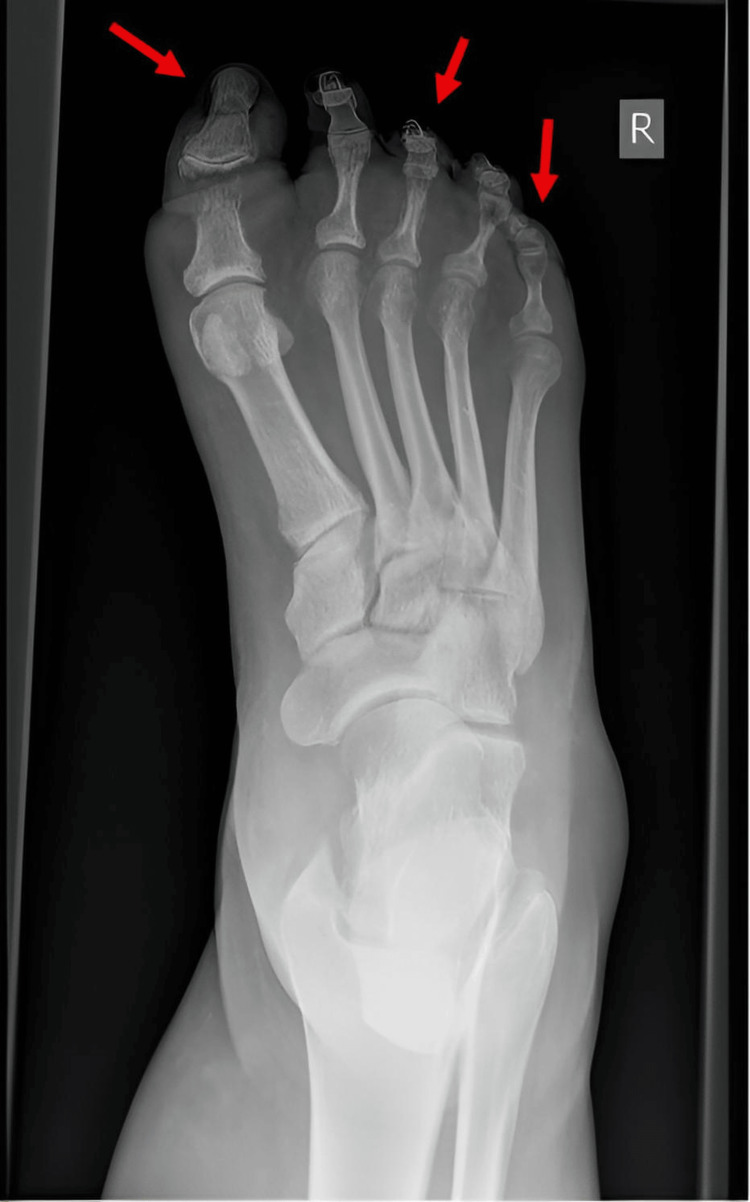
Initial foot radiograph showing osteomyelitis of the great toe with soft tissue gas and periosteal changes in the third and fifth toes.
